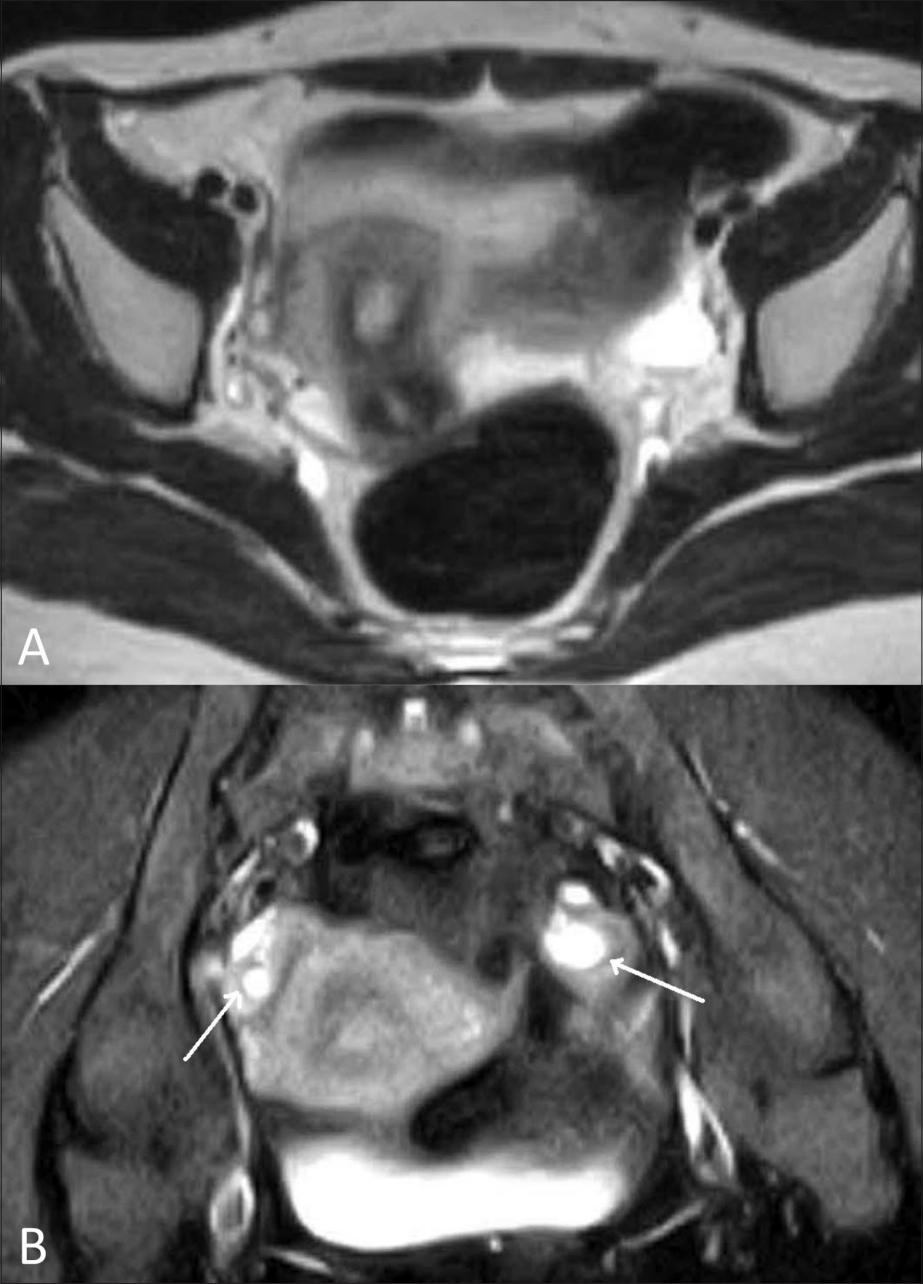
Pelvic inflammatory disease. Axial T2W (A) and oblique coronal fat-suppressed T2W (B) MRI images show pelvic inflammatory disease with resorbed tubes, an infected broad ligament, and thickwalled ovaries (white arrows)
